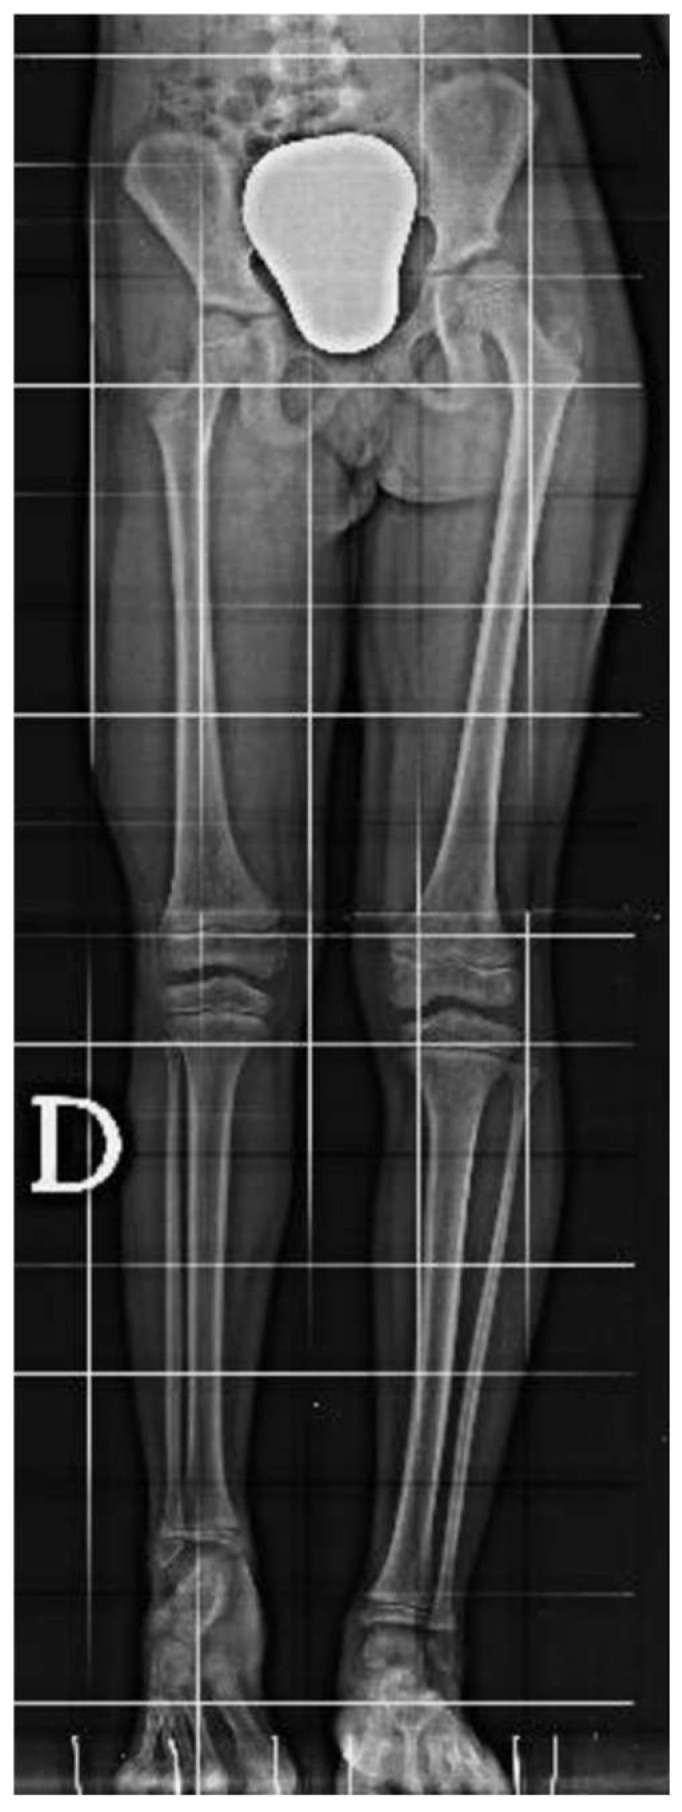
Long-standing plain film radiographs of the patient showing an acquired leg length discrepancy of 75 mm.
